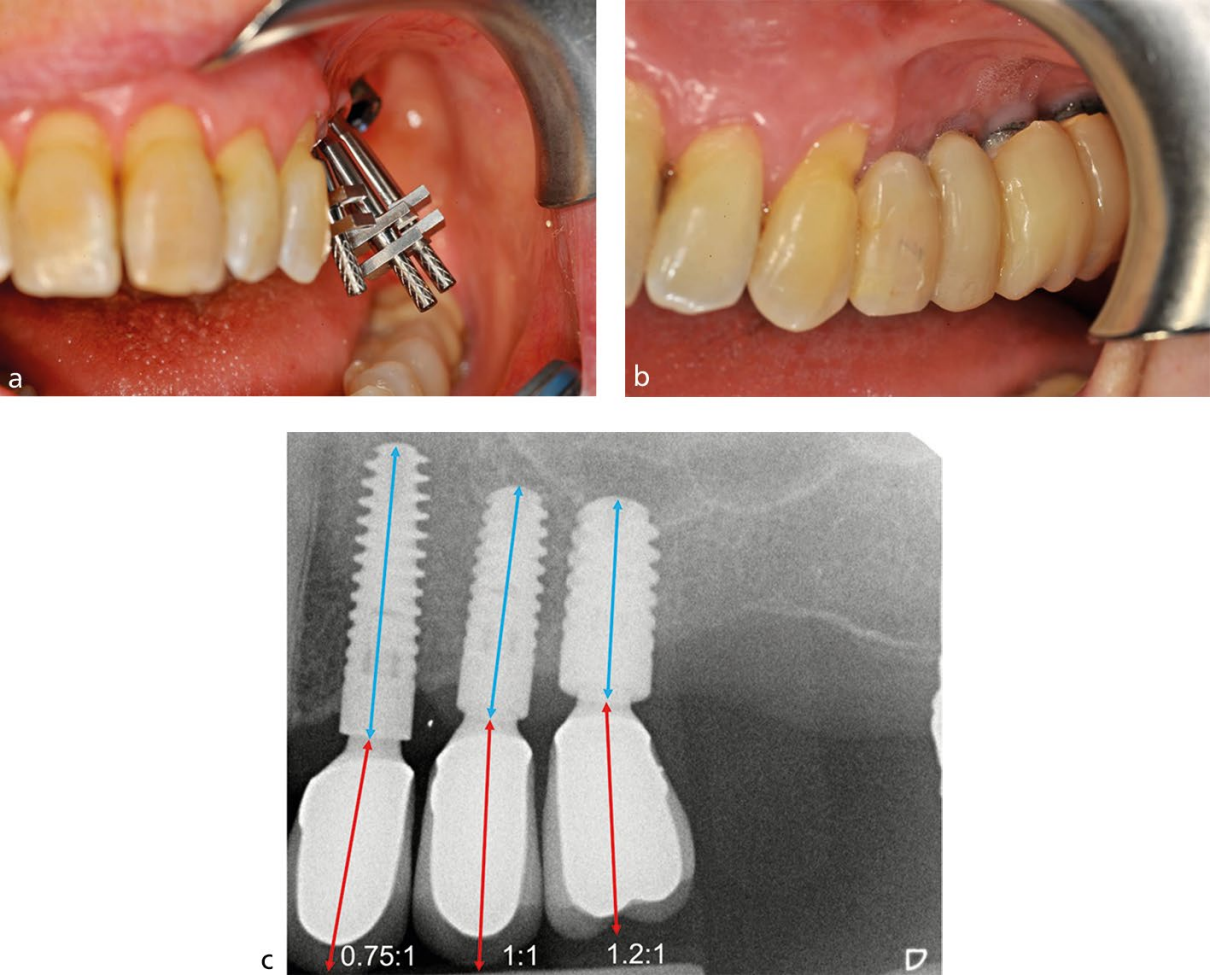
a) Buccally angled implants placed to overcome severe (6 mm) horizontal ridge resorption to achieve ideal crown position and ideal occlusal relationships. b) Try-in of temporary prosthesis. Note the compromised CRP and presence of buccal cantilever to achieve the ideal posterior occlusal relationship. c) IOPA radiograph showing ideal C:Is of ≤1:1 (24, 25) and moderately compromised C:I between 1:1 and 2:1 (26)
